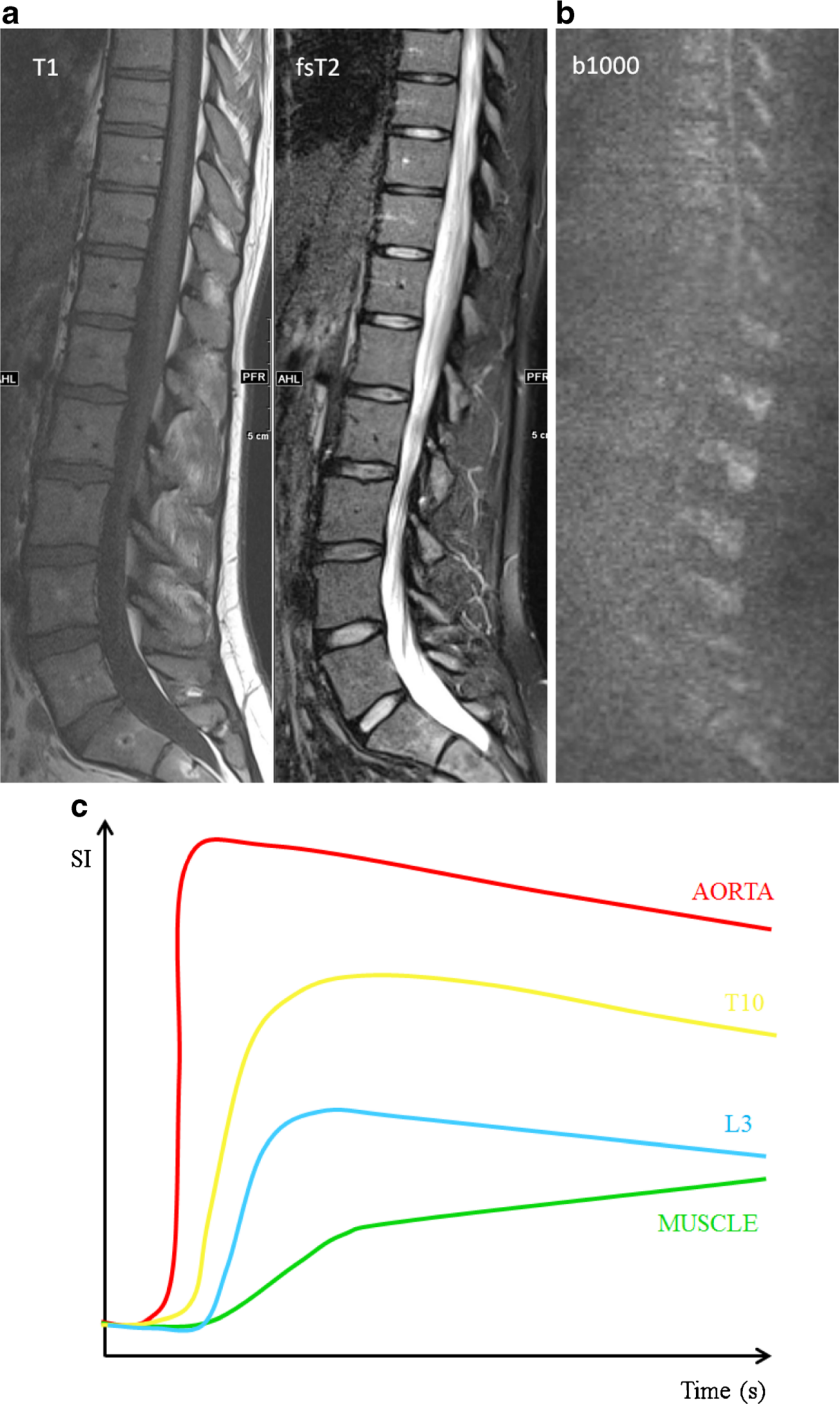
MR images of healthy young subject with normal red bone marrow. a The bone marrow appears hypointense on T1-weighted images (*left*), without focal lesions and with a corresponding high signal intensity on fat-suppressed T2-weighted images (*right*). b There is a high signal intensity on b1000 images due to the low amount of fat cells, high cellularity (hematopoietic cells) and high amount of water. c The vascularization and perfusion are also high (TIC type 4), with an early wash-out of contrast medium
